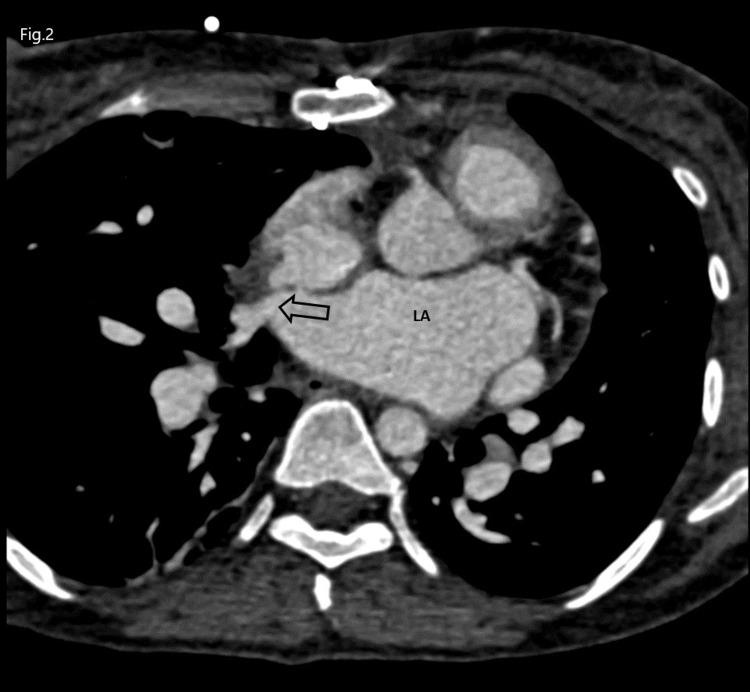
Contrast-enhanced CCT in axial four-chamber view showing right pulmonary vein opening in the left atrium after rerouting (thick black arrow) CCT: Cardiac Computed Tomography, LA: Left Atrium
